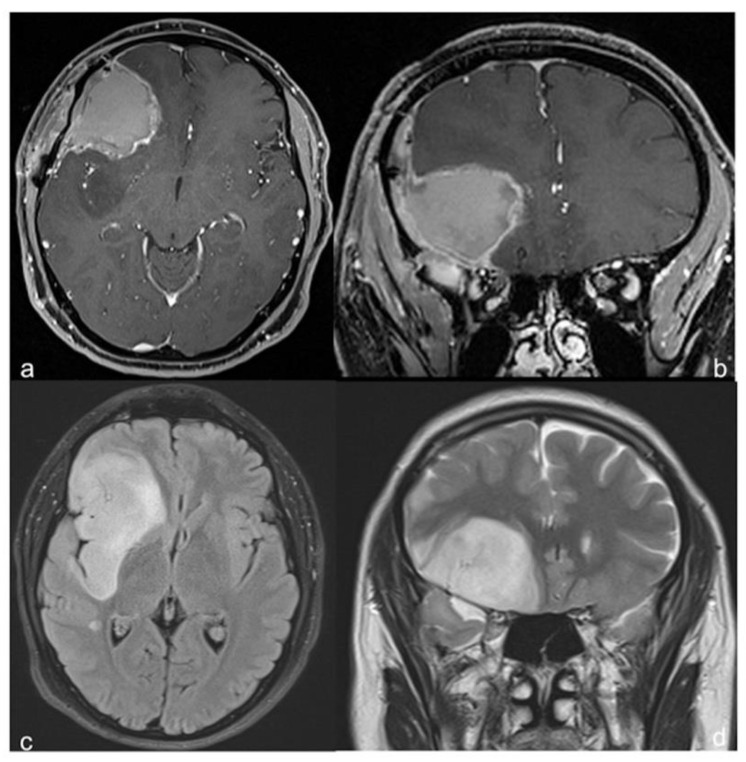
Postoperative brain MRI. Axial (a) and coronal (b) postcontrast T1-weighted, and axial (c) and coronal (d) T2-weighted, sequences; large resection of the frontal supra-sylvian tumor component.
